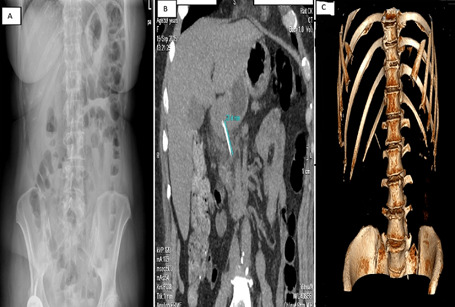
abdominal radiography showed no abnormalities (A); computed tomography (CT) scan of the abdomen revealed a linear, hyperdense, foreign body (B,C)
